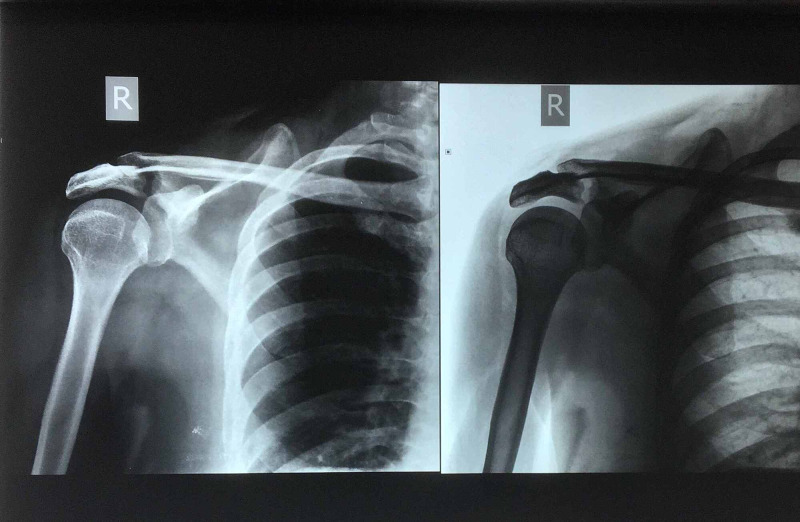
X-ray at five weeks showing displacement and subacromial space compromise
